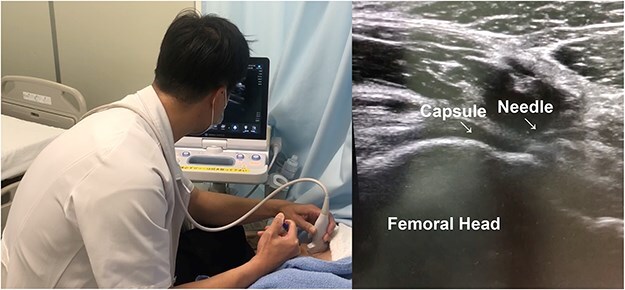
Ultrasound-guided PRP or HA injection for the hip joint using the anterosuperior parasagittal approach.
